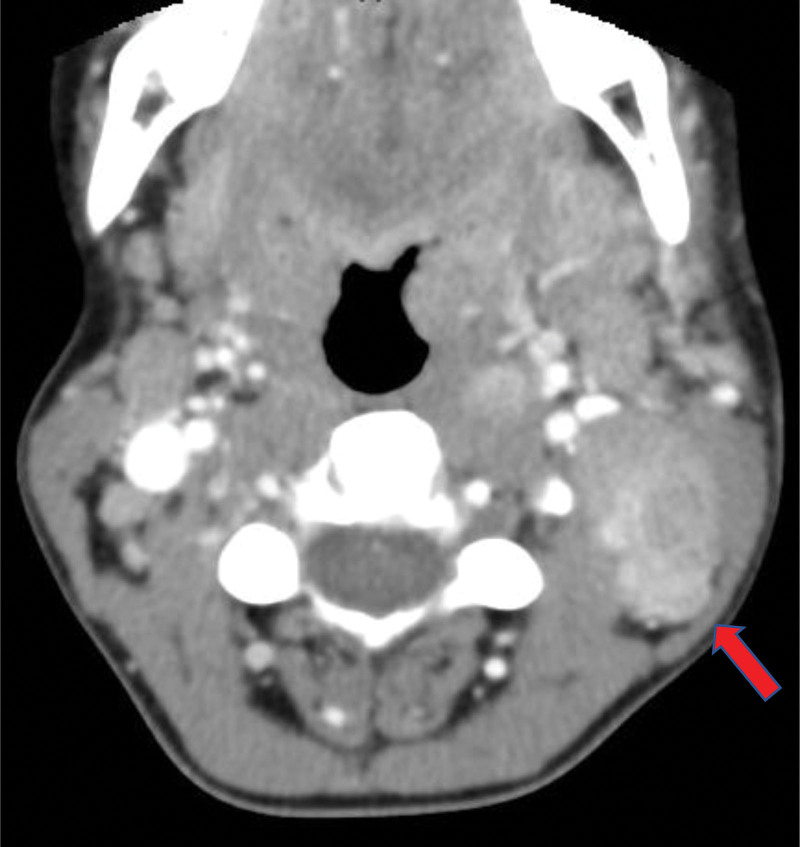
Contrast enhanced cervical CT scan showing enlarged cervical lymph nodes (red arrow) in the left para carotid space, compressing on the posterior wall of the left internal jugular vein, showing heterogenous parenchyma.
